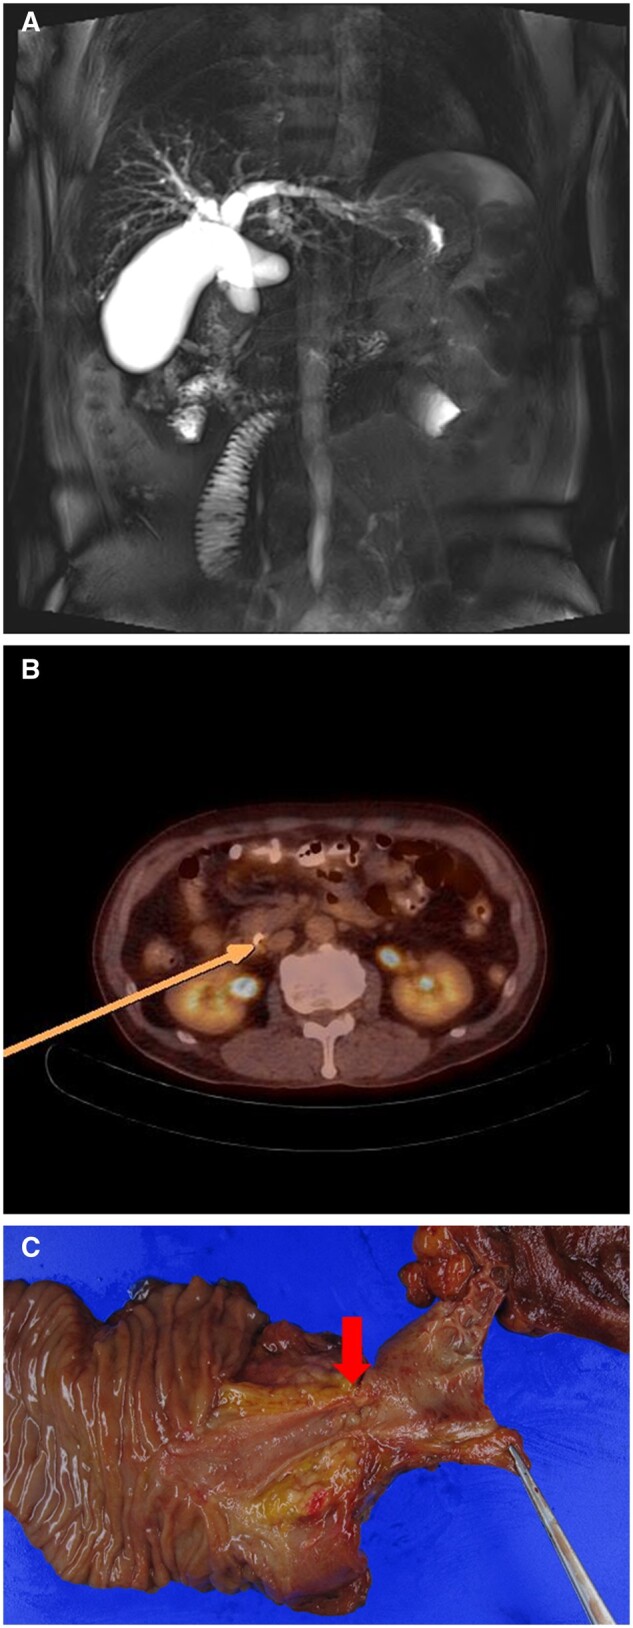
Radiologic findings and macroscopic appearance of the case of a small-cell neuroendocrine tumor in the distal common bile duct (CBD). (A) Magnetic response cholangiopancreatography (T2 projection image) shows the abrupt cut-off sign in distal CBD that strongly suggests cancer. (B) Positron emission tomography-computed tomography demonstrated the mild focal uptake (arrow, SUVmax 2.7) in the distal CBD. (C) The macroscopic appearance showed the narrowed lumen of the distal bile duct (arrow).
